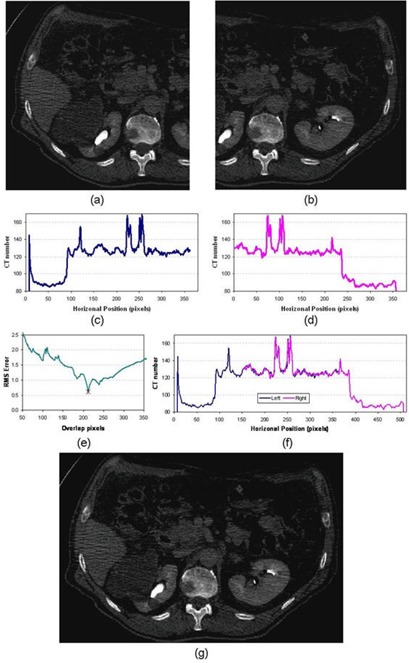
Double partial CT approach on a patient study: (a) left CT slice; (b) the corresponding right slice; (c) the left line profile; (d) the corresponding right line profile; (e) the matching errors; (f) the matching line profiles; and (g) the reconstructed complete CT slice.
